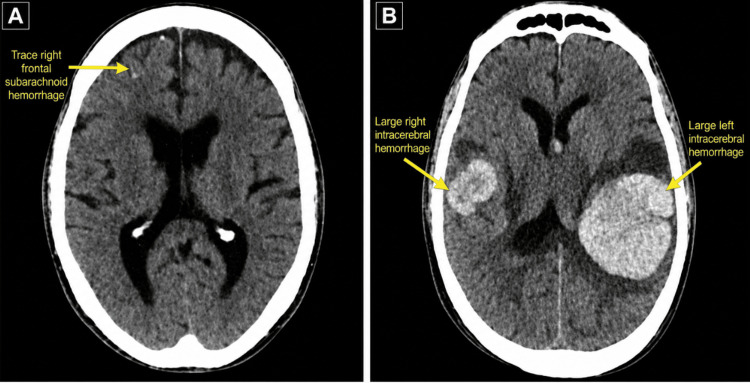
Serial CT head imaging demonstrating progression of intracranial hemorrhage Serial CT head imaging demonstrating progression of intracranial hemorrhage. (A) Trace subarachnoid hemorrhage identified on initial CT imaging on hospital day 0 (yellow arrow). (B) Extensive bilateral intraparenchymal hemorrhages with surrounding edema and mass effect on repeat CT imaging on hospital day 6 (yellow arrows) secondary to hemorrhagic septic emboli from *Rothia mucilaginosa* prosthetic valve endocarditis.
